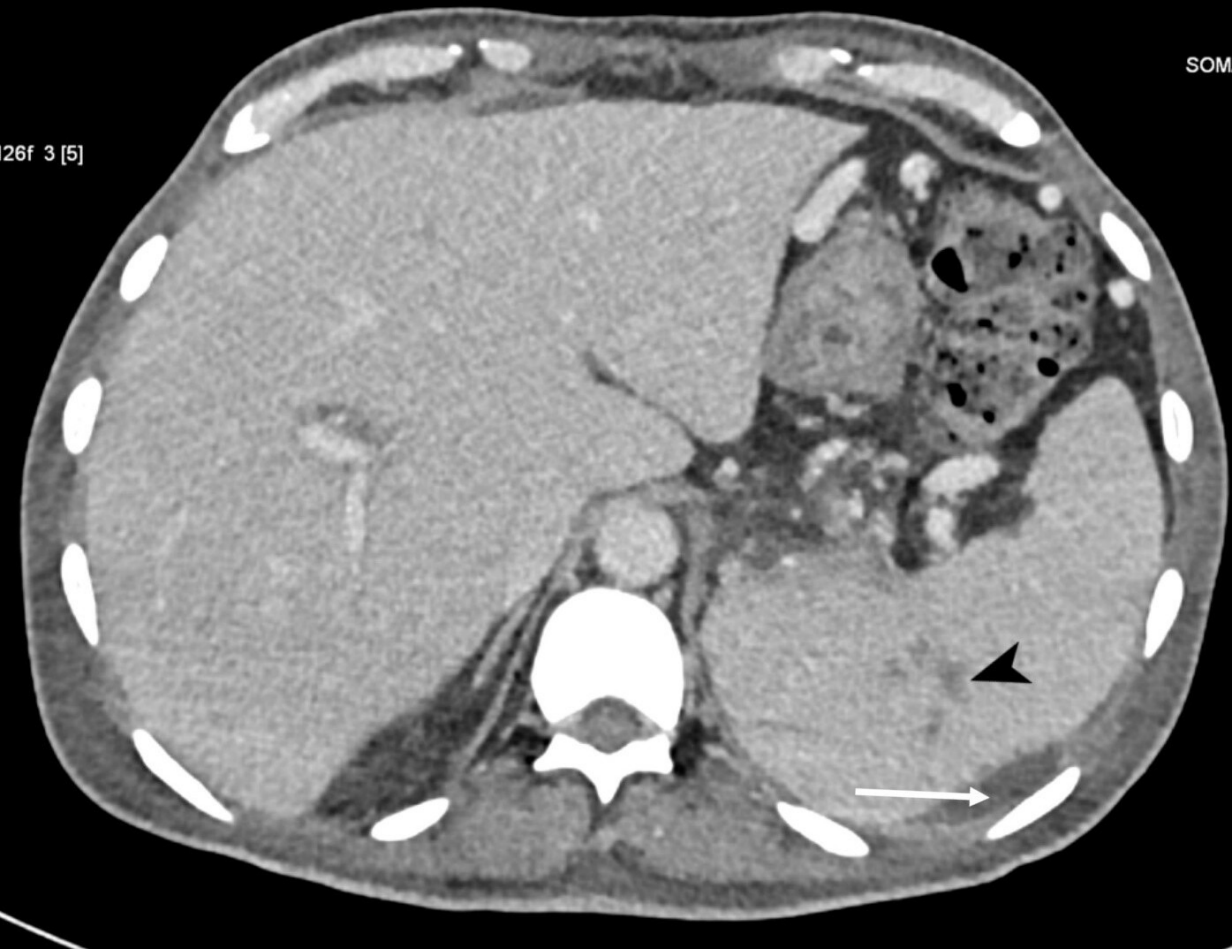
Computed tomography image (axial view) showing multiple small splenic abscesses (arrowhead) with minimal perisplenic fluid collection (arrow) that resulted from the abscess rupture.
